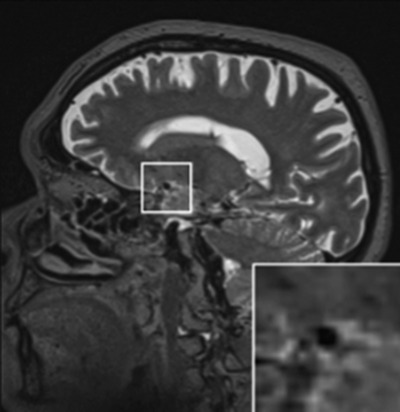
Example of a T2‐weighted MRI sagittal scan of the middle cerebral artery (MCA). The white box indicates the zoomed area showing the lumen of the M1 portion of the MCA
